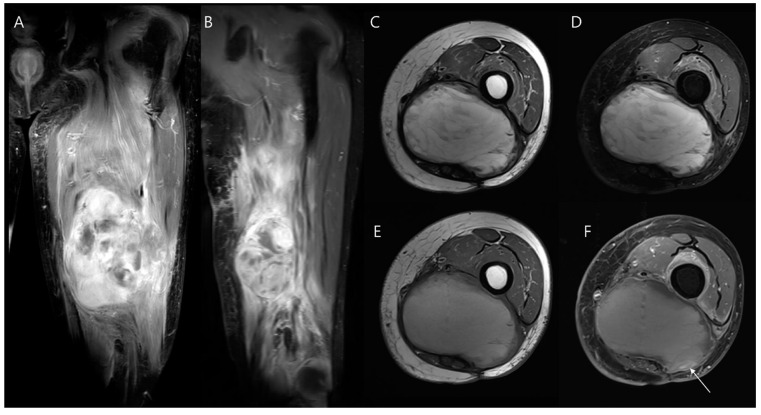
Chronic expanding hematoma after two wide excisions for myxofibrosarcoma in the thigh. (A) Initial coronal T1-weighted fat-suppressed enhanced MR image shows a large soft tissue mass with areas of necrosis and nodular enhancement, necessitating a wide excision. (B) Follow-up coronal T1-weighted fat-suppressed enhanced MR image shows a recurrence of the mass with a discrete margin and round shape, leading to a second wide excision. One year post-surgery following the final wide excision, axial (C) T2-weighted, (D) T2-weighted fat-suppressed, (E) T1-weighted, and (F) T1-weighted fat-suppressed enhanced MR images show a well-defined, hemorrhagic, mass-like lesion with peripheral nodular enhancement (arrow in (F)) within the surgical site. This lesion exhibited slow growth, and debulking surgery was performed to address the chronic expanding hematoma.
